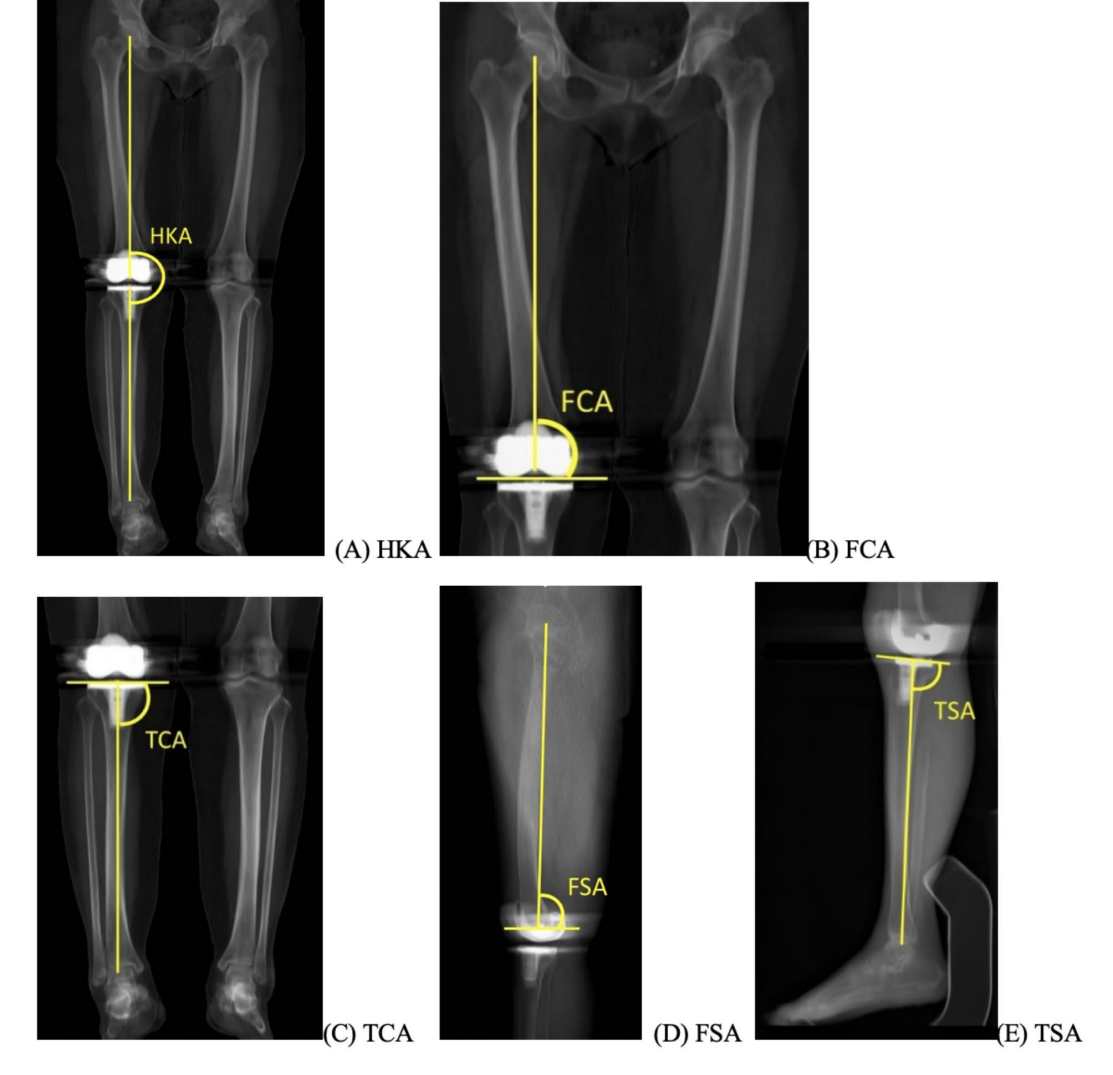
These photos depict radiographic measurements with CT scans in the coronal plane to evaluate HKA (A), FCA (B), and TCA (C), and in the sagittal plane for FSA (D), and TSA (E)
